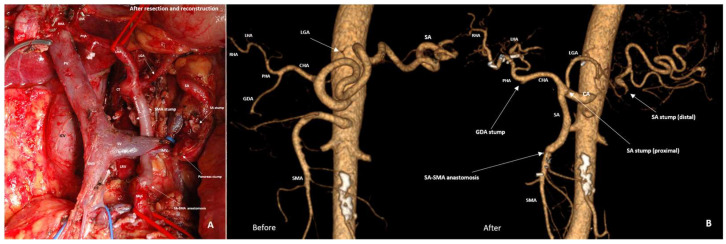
Pancreaticoduodenectomy with SMA and SMV resection, spleen- and distal pancreas-preserving resection and rotation of the splenic artery (SA) for SA-SMA anastomosis for PHDAC with SMA and SMV involvement in a 65-year-old woman. (A) An operating field after completion of the resection stage. The SA is rotated clockwise and anastomosed with the SMA. Resection of the posterior wall of the SMV. The SV is pulled down by the blue vessel loop to expose the SMA stump. (B) CTA shows the sources of blood supply to the spleen and pancreatic tail from the branches of the LGA after surgery, the diameter of which increased by 1.8 times (B), increasing the VBF through the LGA by 10.5 times.
